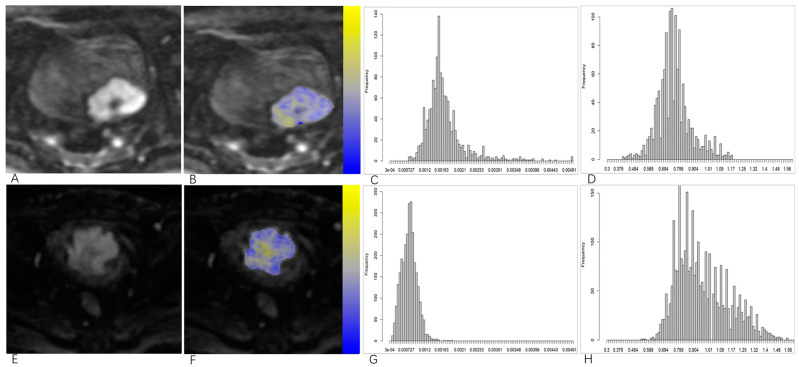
Upper row: a 63-year-old man with bladder cancer (papillary urothelial carcinoma, category T1, low grade): (A) axial DWI images; (B) regions of interests (ROIs) of the tumor area; (C) volumetric MD histogram; (D) volumetric MK histogram. Lower row: a 74-year-old man with bladder cancer (papillary urothelial carcinoma, category T2, high grade): (E) axial DWI images; (F) regions of interests (ROIs) of the tumor area; (G) volumetric MD histogram; (H) volumetric MK histogram.
